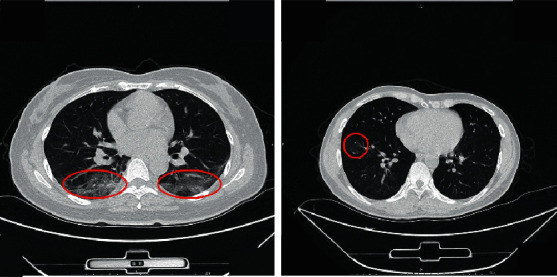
The lesion area of COVID-19 on the CT images.
